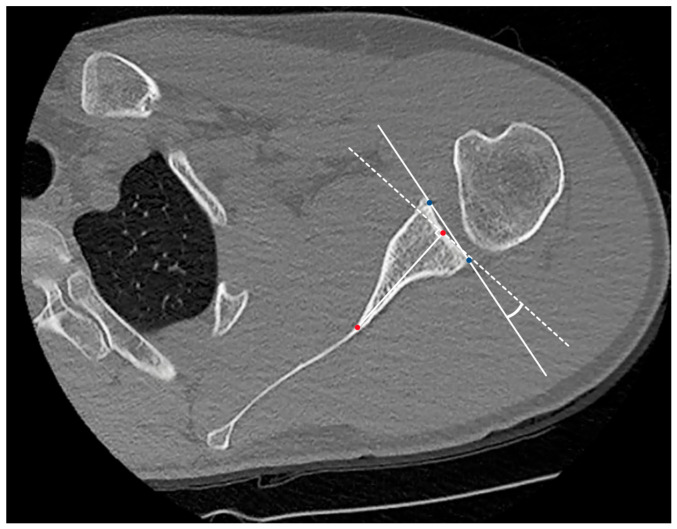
The first axial CT image in which the scapular body was completely visualized was selected for measurement using the Vault method. The glenoid line (solid line connecting the blue dots) connects the anterior and posterior glenoid margins. The vault line (solid line connecting the red dots) extends from the apex of the scapular vault to the midpoint of the glenoid fossa. Glenoid version was measured as the angle between the glenoid line and the line perpendicular to the vault line (dashed line)
